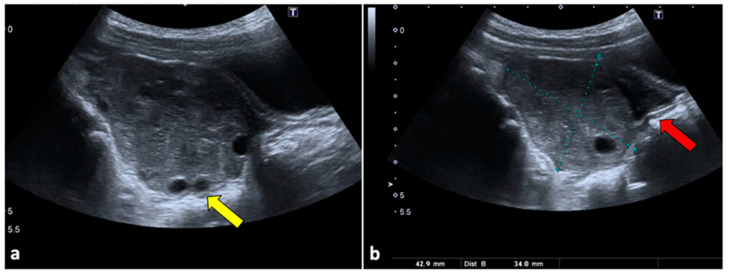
Ovarian torsion in an eight-year-old patient presenting at the emergency department with aching right pelvic pain for a few hours. Transabdominal US examination (a,b) shows that the right ovary is grossly enlarged (long axis: 42.9 mm; short axis 34 mm) and abnormally located in the midline. The ovary appears slightly echogenic, with many small cysts at the periphery (yellow arrow). Small volume free fluid with tiny echoes is present in the pelvis (red arrow). During the Doppler US evaluation, no vascular flow was noticed (not shown).
